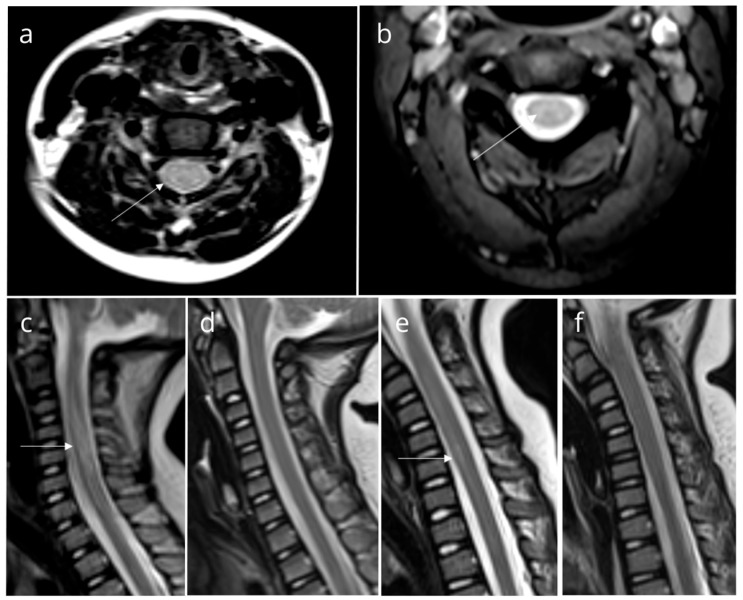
Magnetic resonance imaging (MRI) findings in patients. Axial (a) and sagittal (c) T2-weighted MRI of Patient 2, axial T2-weighted MRI of Patient 3 (b), and sagittal T2-weighted MRI of Patient 6 (e) show high-intensity signals suggestive of myelitis (white arrow). Follow-up images of Patient 2 (d) and Patient 6 (f) reveal normal findings. All other patients had similar findings.
